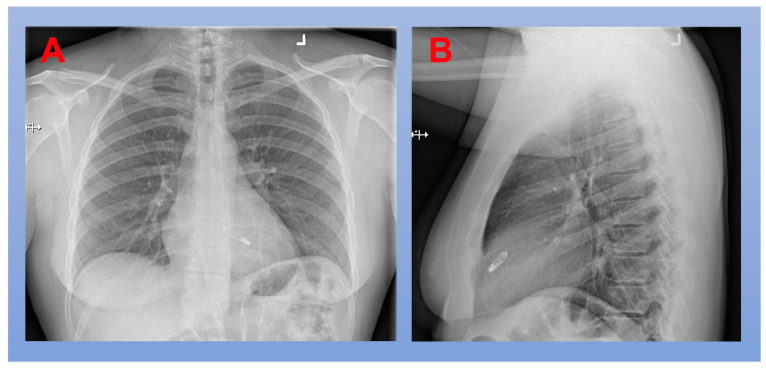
Chest X-Ray After Implantation of a Leadless Pacemaker. (A) PA projection; (B) lateral projection.
